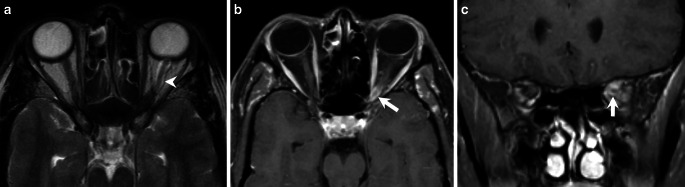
Axial T2-weighted MRI from March 2008 (a) revealed a dilatation of the dural space along optic nerve (ON) sheath (arrowhead). Axial (b) and coronal (c) T1-weighted contrast-enhanced fat-suppressed (T1 C+ FS) images demonstrated a concentric, enhancing lesion encasing the compressed, non-enhancing ON, producing the characteristic “tram-track” and “doughnut” appearances (arrows)
